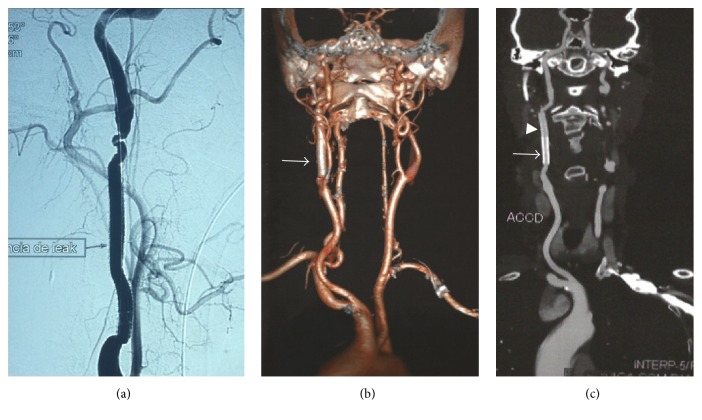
(a) Intraoperative angiography after second stent graft deployment, patent carotid, and leak absence; (b) one-year CT control, arrow points to the stent grafts, no signs of stents occlusion; (c) one-year CT control, arrow points to the first deployed stent grafts and arrow head points to the second stent graft.
